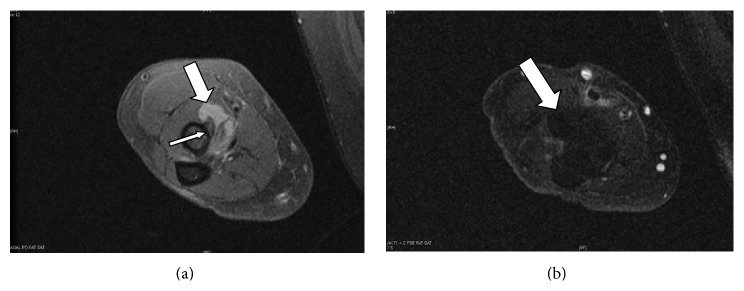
(a) MRI axial T1 weighted before IV gadolinium. Hyperintense signal showing the bursa (Fat arrow) and biceps tendon (thin arrow). (b) MRI axial T1 weighted after IV gadolinium sequences. Bursa (thick arrow).
